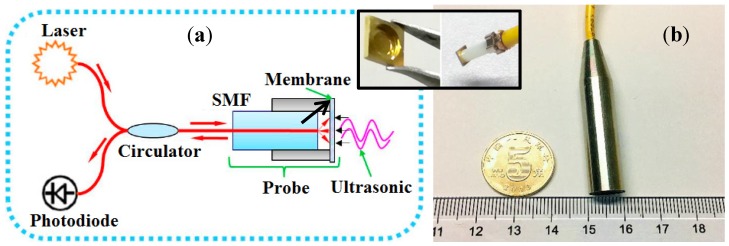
(a) Operational principle for detecting ultrasonic signals by utilizing the MEMS-on-fiber sensor. (b) Picture of a packaged sensing probe. SMF: single-mode fiber.
